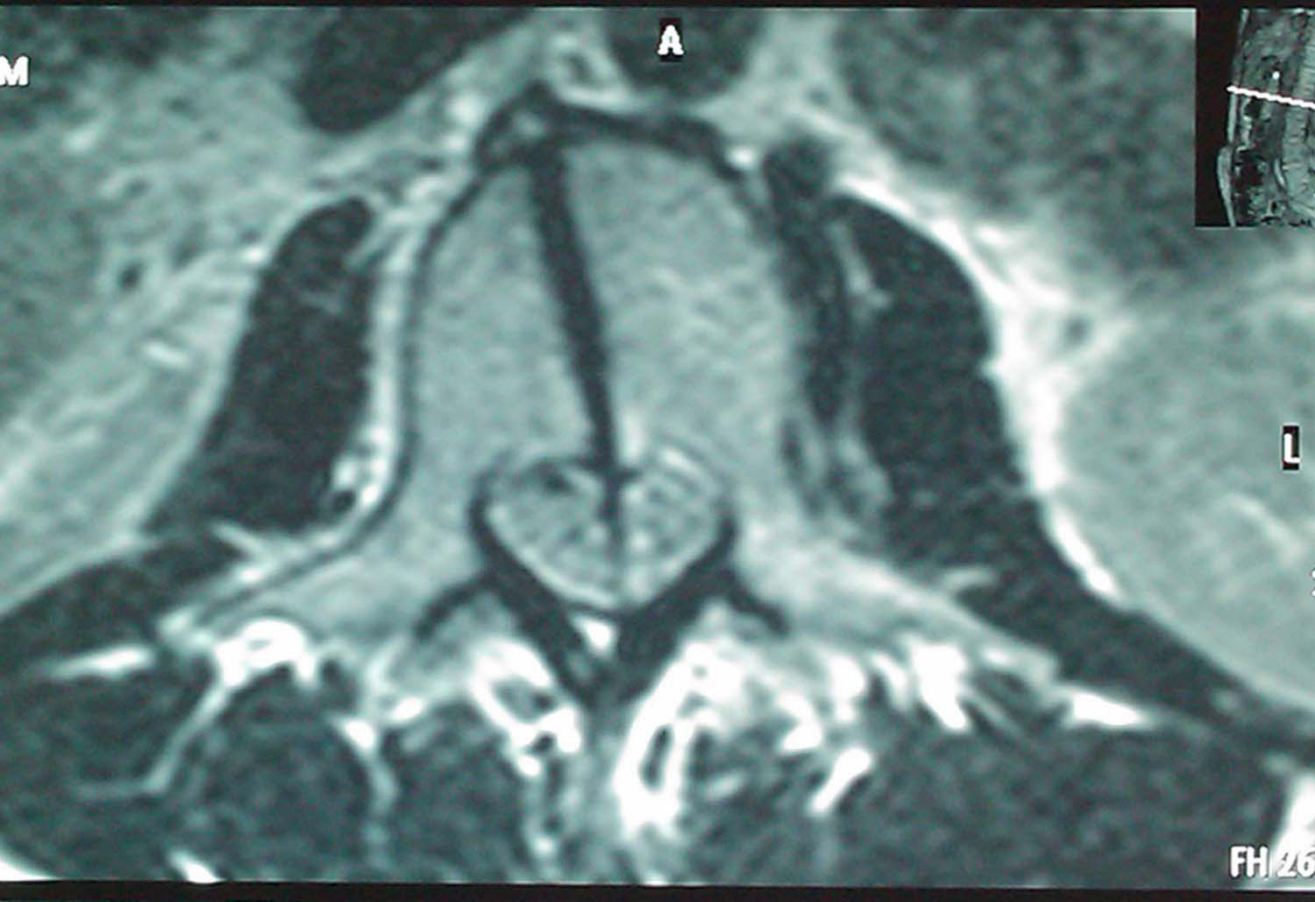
MRI scan of the lumbar region that indicates a foreign body penetrating the body of the L2 and the spinal canal.
